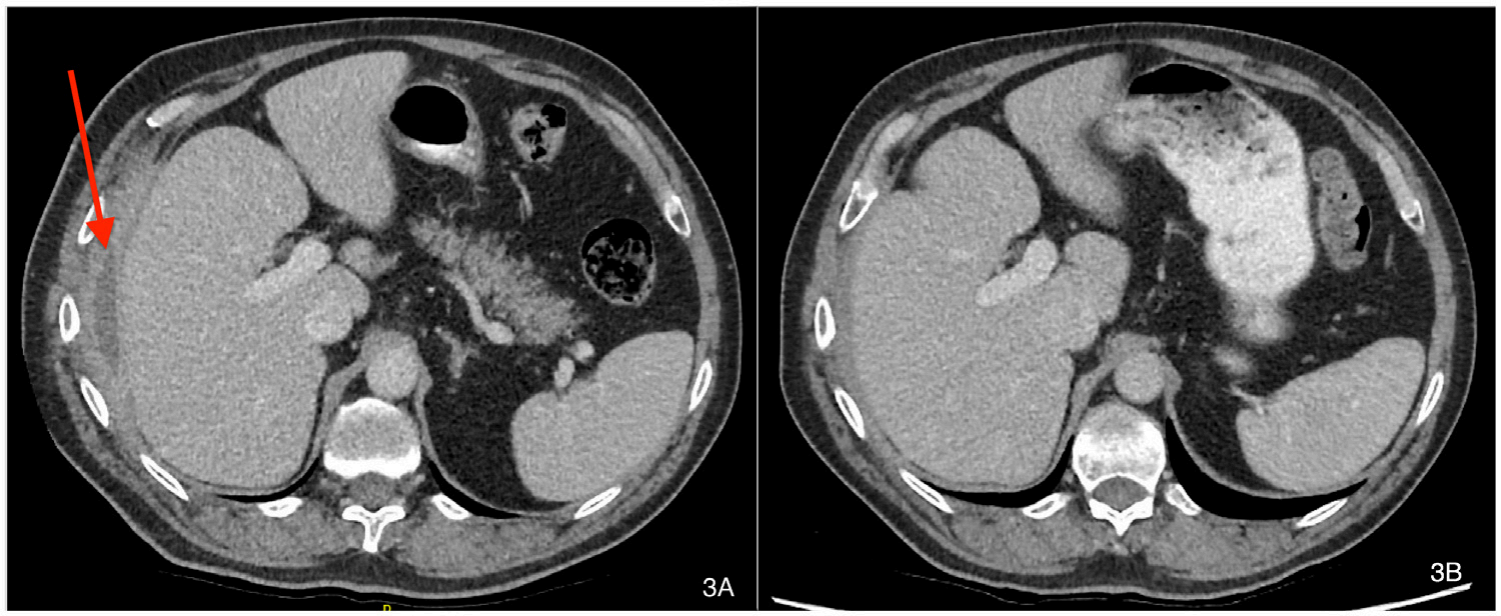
CT imaging before (A) and at 16 weeks (B) of antibiotic therapy (labels added with Mac’s Preview App v11.0).
